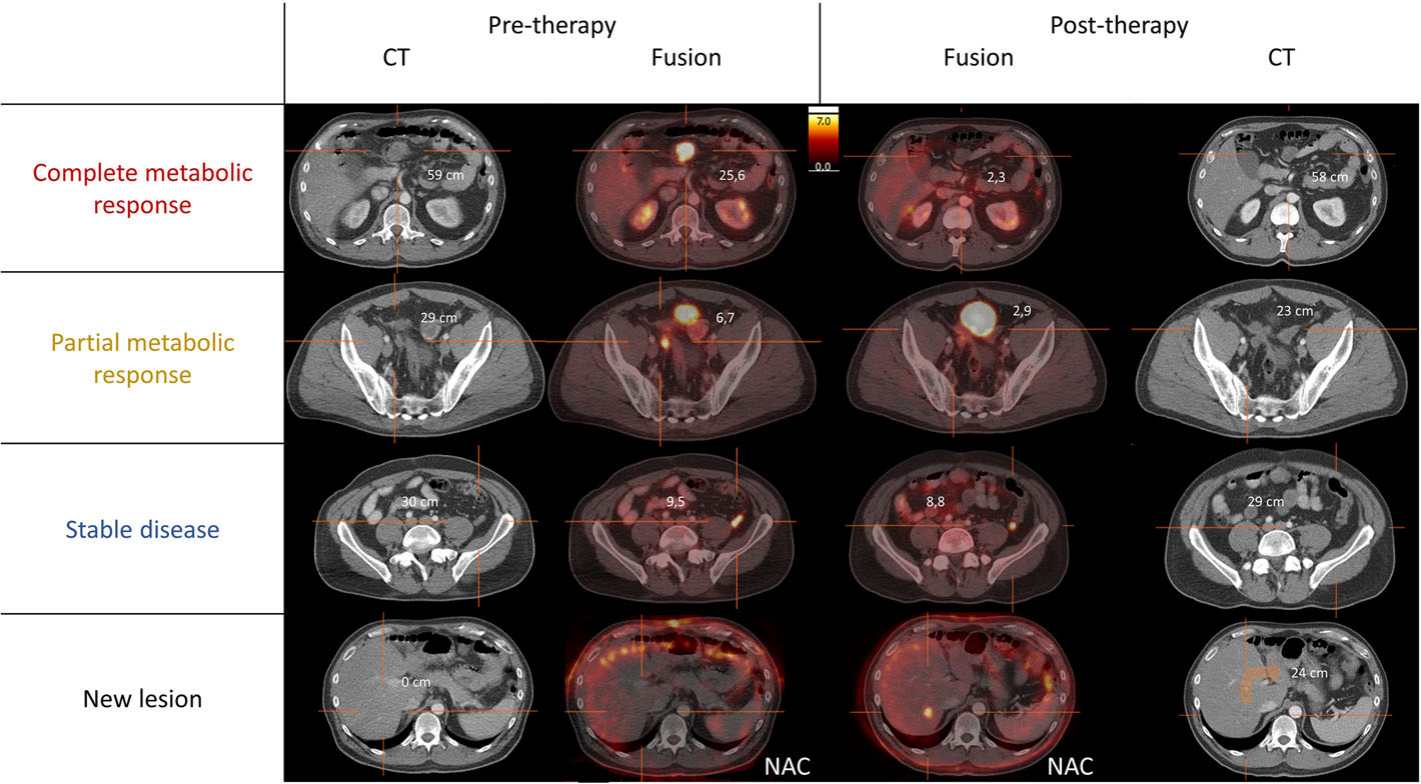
Pre- and post-therapy fused PET/CT and CT images of the different lesions. NAC = non-attenuation-corrected images
